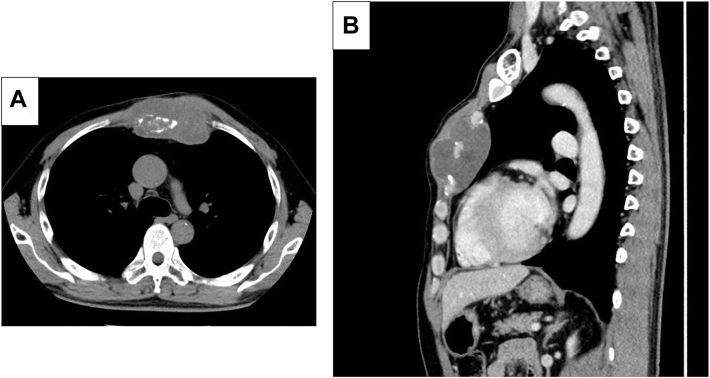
Radiologic findings. (A) Axial and (B) sagittal computed tomographic image showing an 83 × 45 mm sternal mass with a contrast-enhanced edge.
